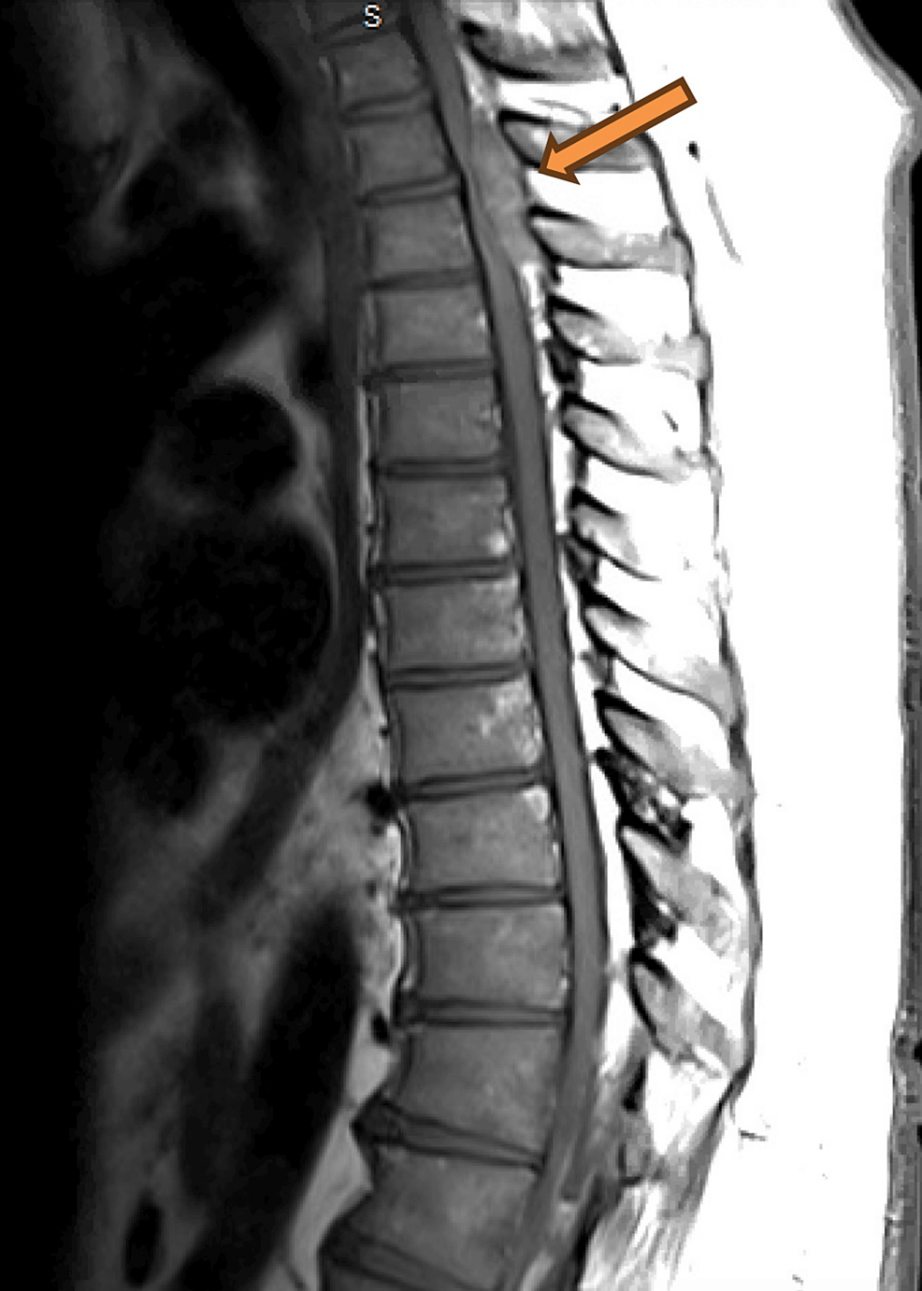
T1-weighted image of MRI thoracic spine with enhancing posterior epidural mass spanning from T1-2 to T3-4.
